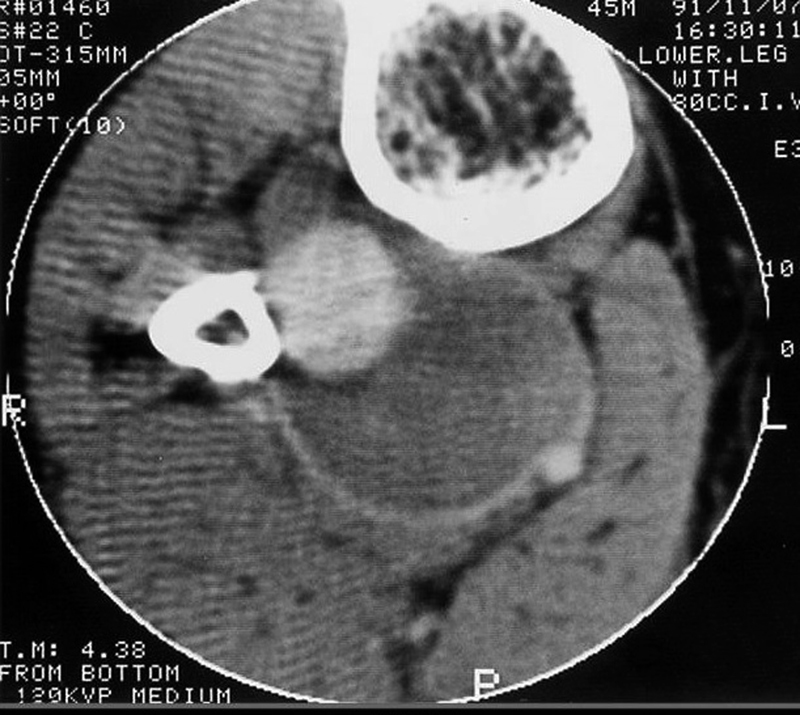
Contrasted CT scan showing the actual size of the aneurysm with its pseudocapsule being presented as rim enhancement. The patent part of the aneurysm is demonstrated as a hyperdense mass compatible with fresh blood which is surrounded by isodense area compatible with old clot. An artifact may be due to the missile fragment hidden behind the bone.
